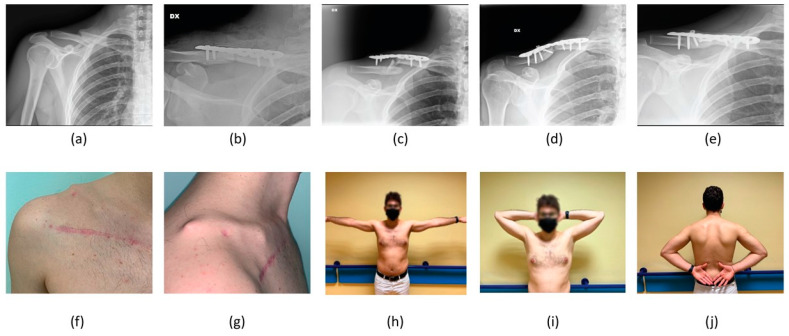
A 27-year-old male patient underwent surgical treatment twice for an AO A2 right ADMCF. Clavicle radiographic image at (a) the first time of patient presentation in ER, (b) immediate post-operative period, (c) after a second presentation in ER showing lateral screw loosening and dorsal displacement of the plate 4 months after the operation, (d) immediately after the second osteosynthesis and (e) at last follow up of 30 months. Clinical images of the patient presentation in ER for the implant failure showing (f,g) considerable swelling in the supraclavicular region. Last follow-up clinical images showing good range of patient movement in abduction (h), extra-rotation (i) and intra-rotation (j) with the plate still on site.
